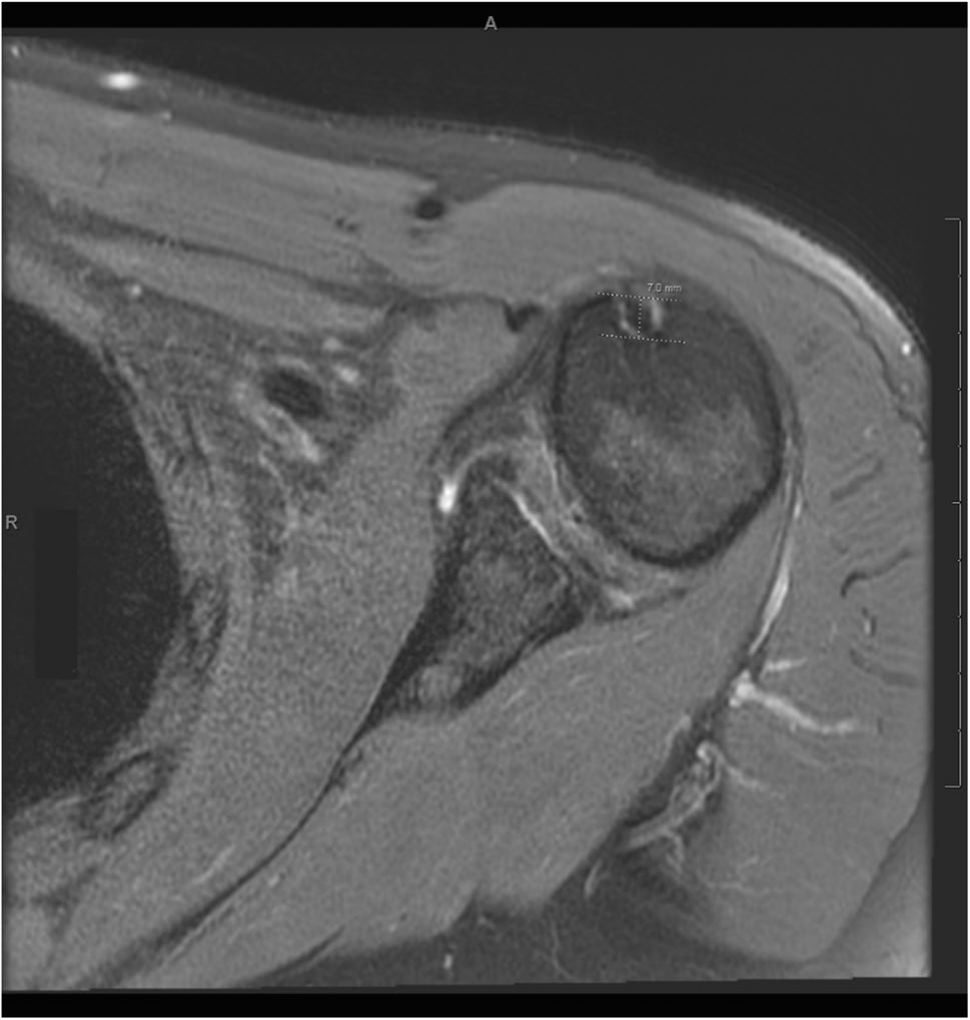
Measurement of biceps groove depth on axial MRI. A line was drawn from the greater tuberosity to the lesser tuberosity at the midportion of the groove. A parallel line was drawn at the deepest part of the groove, followed by a perpendicular line to measure the deepest part of the groove
